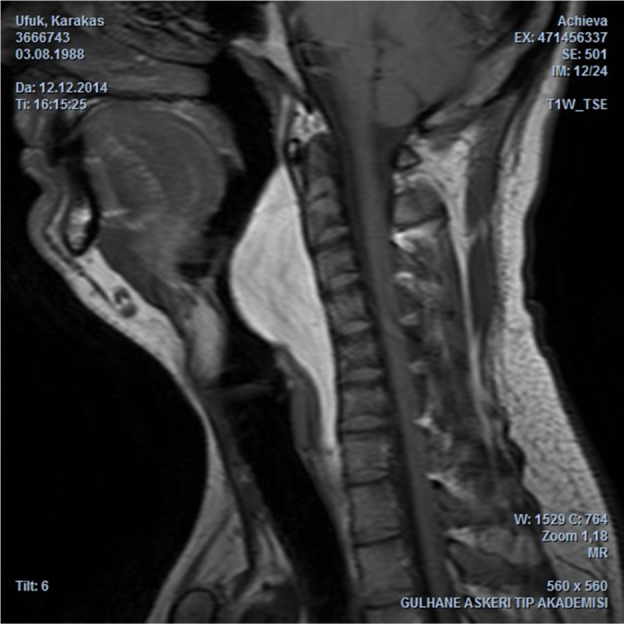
Sagittal T1-weighted MRI depicts the hyperintense retropharyngeal mass lesion similar to fat.
